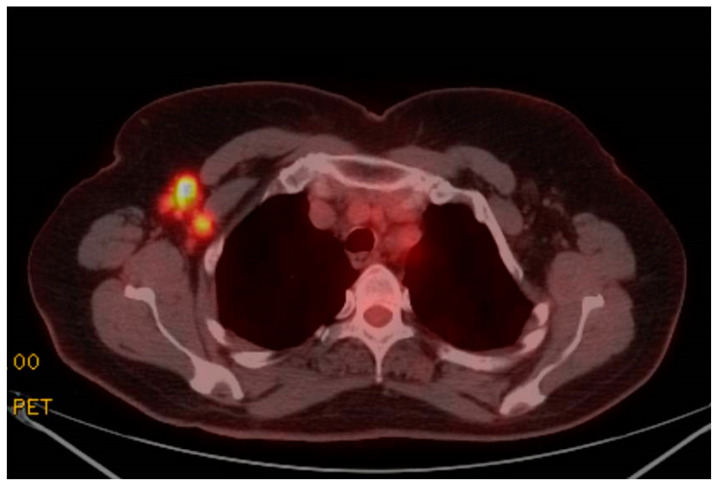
PET/CT imaging shows increased FDG avidity in right axillary lymph nodes.
